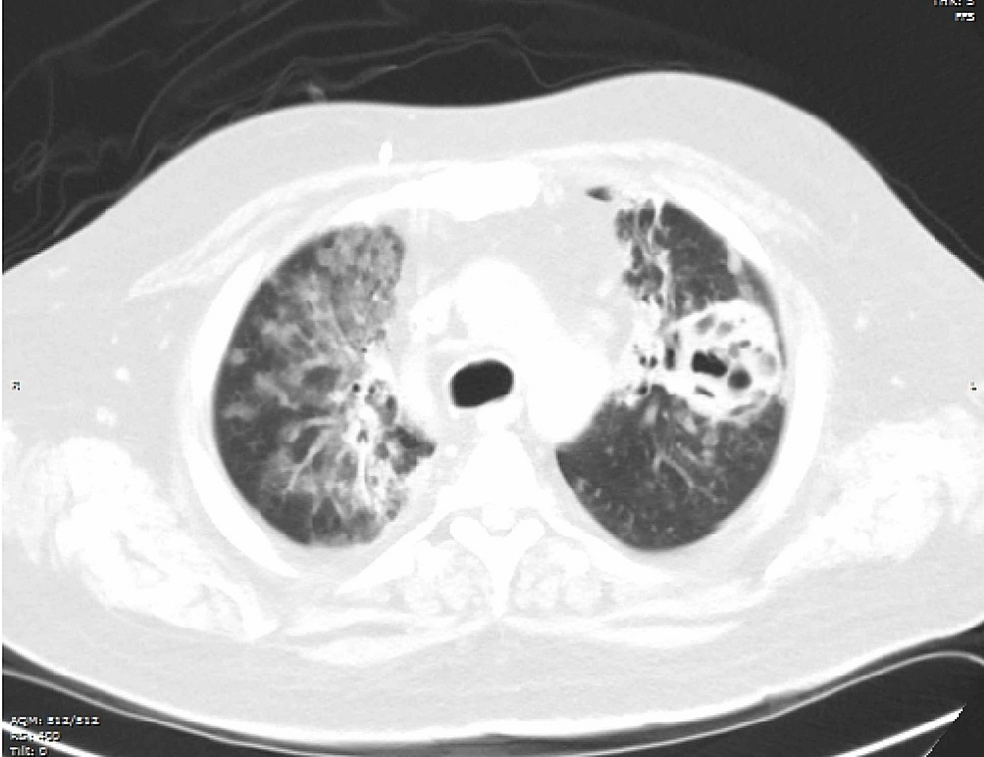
CT scan of the chest without contrast showing pulmonary cavities in a patient with SCLC. CT, computed tomography; SCLC, small cell lung cancer
